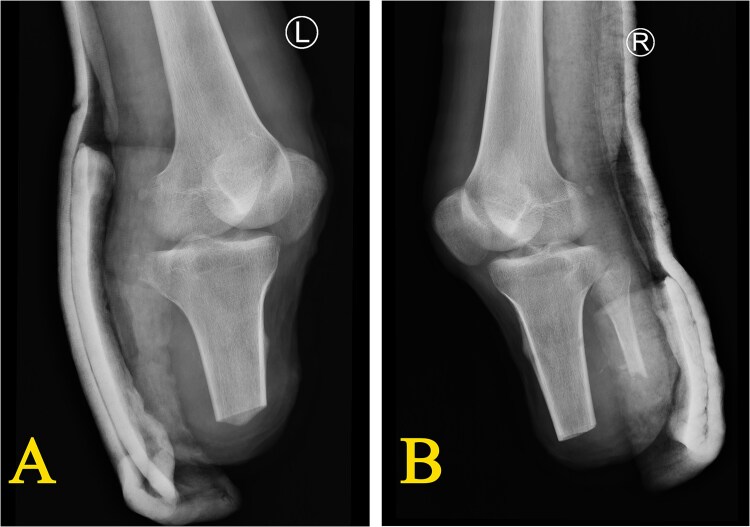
X-ray after bilateral lower limb amputation (D34) (A) post-operative condition of the left calf after local flap transfer repair, showing near complete healing; (B) post-operative condition of the right calf after debridement with vacuum sealing drainage and application of a cast for external fixation.
